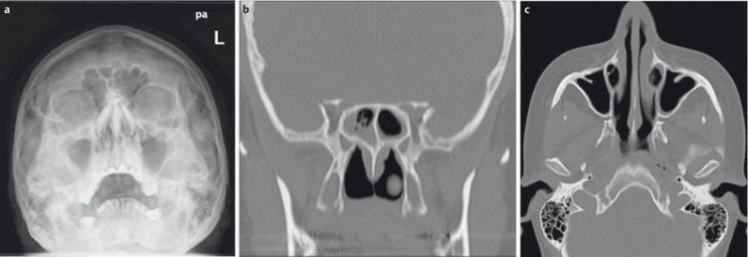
53-year-old patient with leukemia prior to radiation therapy. a) Conventional radiograph of the paranasal sinuses; soft tissue swelling within the right maxillary sinus without air-fluid level. b) CT of the paranasal sinuses of the same patient. The CT was performed 2 hours after plain radiographs. CT shows sinusitis of the sphenoid sinus not detectable on the radiographs. c) CT does not confirm sinusitis of the right maxillary sinus.
